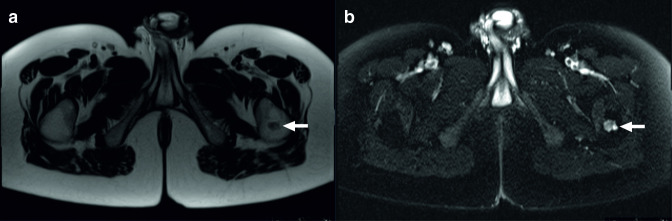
A 30-year-old female with a history of ulcerative colitis and colectomy with ileal pouch-anal anastomosis underwent MRI of the pelvis to evaluate the anastomosis (J-pouch) because of abdominal complaints. At the edge of the scan, a lesion at the left trochanteric region was see as an incidental finding. The 2 cm lobulated intramedullary lesion, visible with low intensity on *T*
